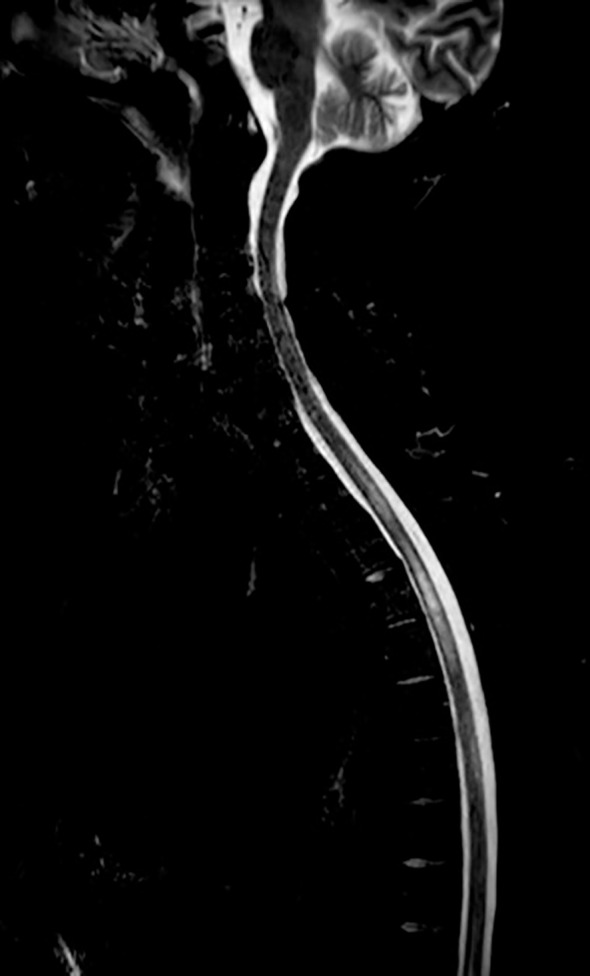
Spinal MRI performed approximately five weeks after the first imaging (refer to Figure 2) - T2-weighted sequences STIR fat suppression.
